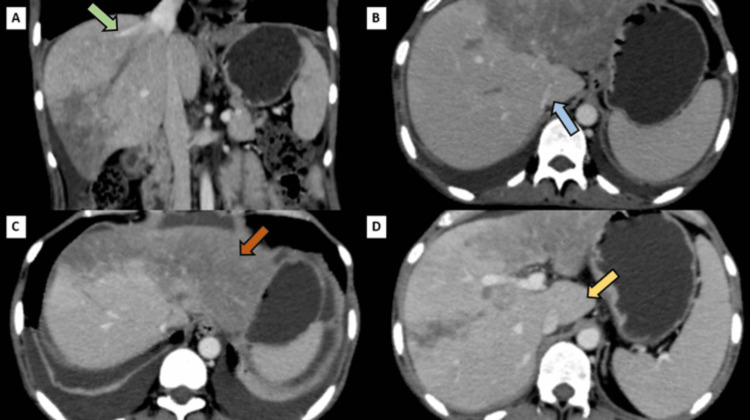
(A) Coronal reformatted CECT image showing complete non-filling of left and middle hepatic veins and partial filing of right hepatic vein(green arrow). (B) Axial section of CECT showing the slit-like intrahepatic IVC (blue arrow). (C) Axial CECT image showing the mottled appearance of the liver (orange arrow). (D) Axial section of CECT showing caudate lobe hypertrophy (yellow arrow). IVC: inferior vena cava; CECT: contrast-enhanced computed tomography
